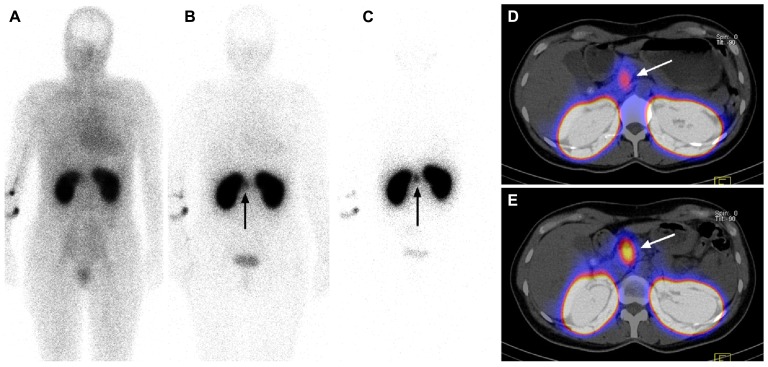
^111^In-DOTA-exendin-4 whole-body planar images (A–C) and ^111^In-DOTA-exendin-4 SPECT/CT images (D,E) from the same patient. Whole-body scans were carried out 20 min (A), 4 h (B), and 3 days (C), and SPECT/CT scans were performed 4 h (D) and 3 days (E) after injection of 97 MBq ^111^In-DOTA-exendin-4. Four hours after injection, there was already a focal ^111^In-DOTA-exendin-4 uptake visible in the head of the pancreas (arrow) on whole-body (B) and SPECT/CT (D) scans. The tumor-to-pancreas-uptake ratio was 1.9 at 4 h after injection (D) and 3.2 at 3 days after injection of the radioligand. The longest residence times of ^111^In-DOTA-exendin-4 were observed in the tumor (arrow) and kidneys (C). Reprinted from Christ et al. (2009), with permission from The Endocrine Society.
